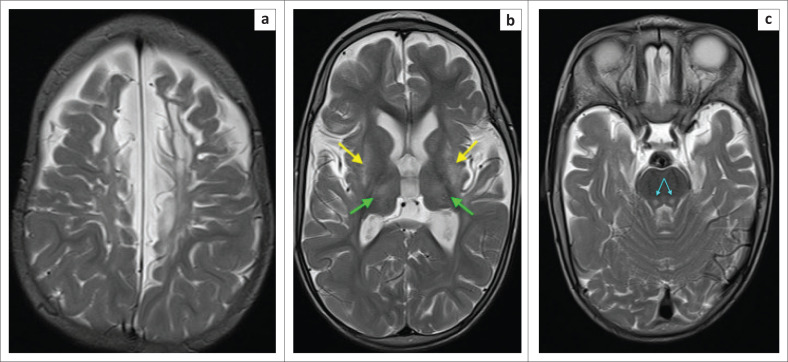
A 2-year-old male child with severe mixed type hypoxic ischemic brain injury. T2-weighted axial images (a) at the level of the centrum ovale demonstrating parasagittal frontal lobe injury with atrophy beyond the central sulcus and paracentral lobule, involving the superior frontal gyri with ulegyria, (b) at the level of the basal ganglia shows the hyperintense signal change at the dorsal putamina (yellow arrows) and ventral thalami (green arrows) and (c) at the level of the superior cerebellar peduncles reveals prominent central tegmental tract (cyan blue arrows) hyperintensity at the dorsum of the pons.
